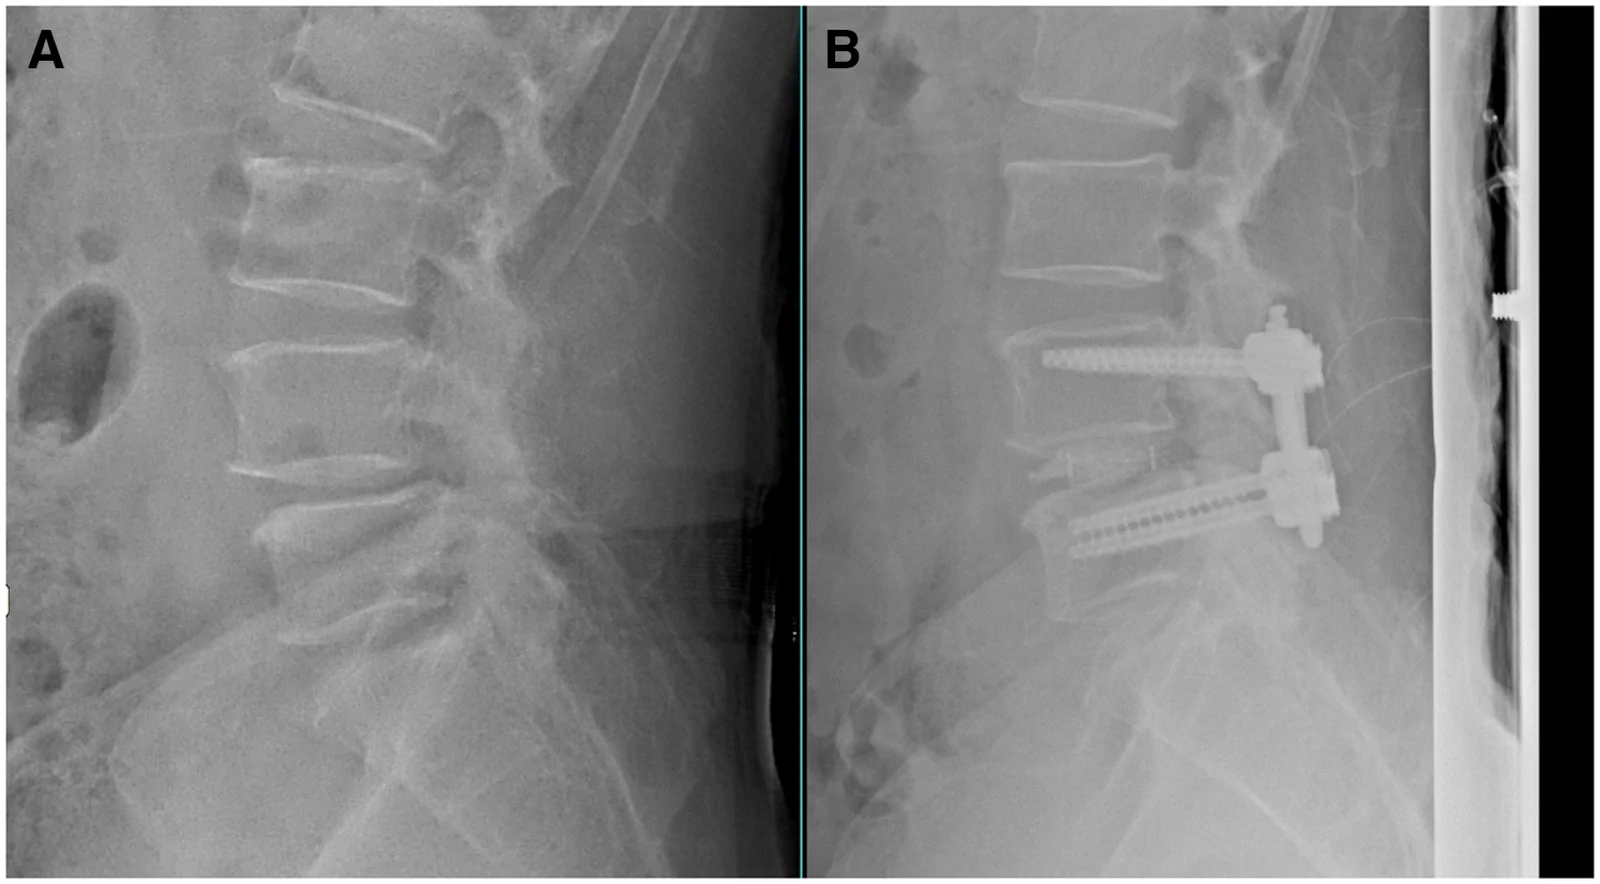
(A) Preoperative lumbar spinal X-ray; (B) postoperative X-ray after L4–5 minimally invasive transforaminal lumbar interbody fusion.
